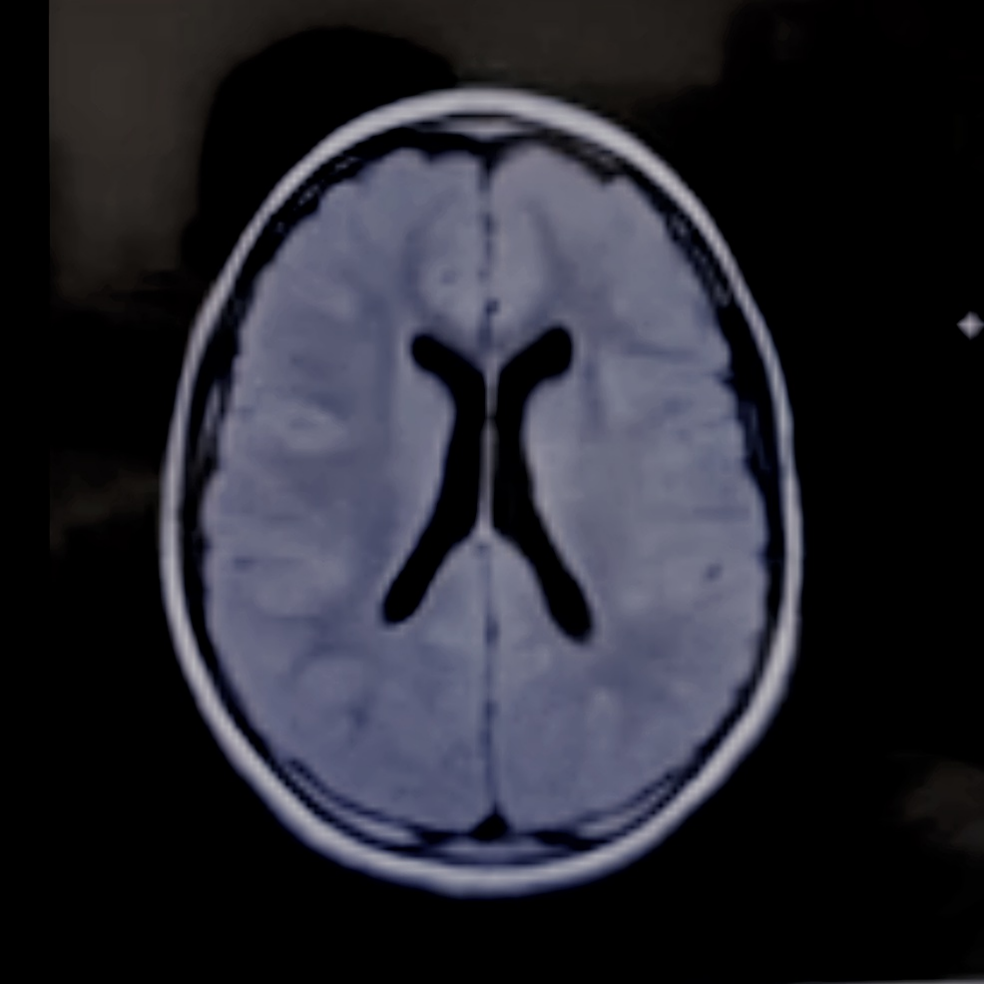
MRI Brain Axial View No evidence of mass effect, midline shift or intracranial hemorrhage. Hypoplastic appearance of the corpus and splenium. No ventriculomegaly.
